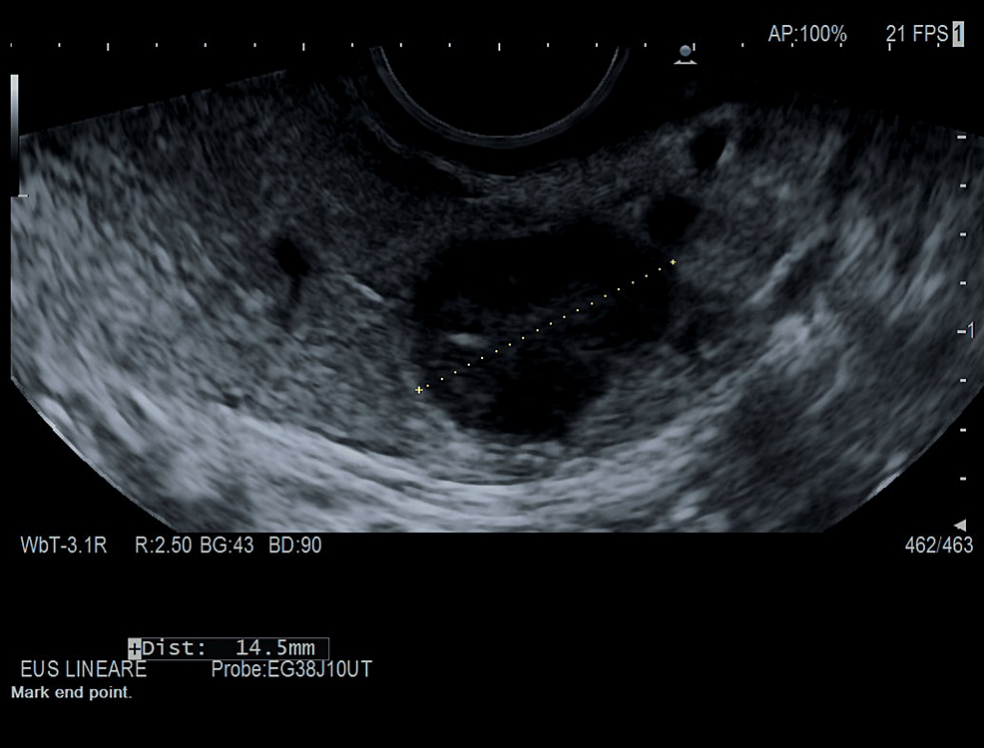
EUS of our patient conducted with EG38-J10UT sector echoendoscope, at the level of the pancreatic isthmus, roundish neoformation, hypoechoic of 13.8 mm × 12.8 of maximum size. EUS: endoscopic ultrasound
